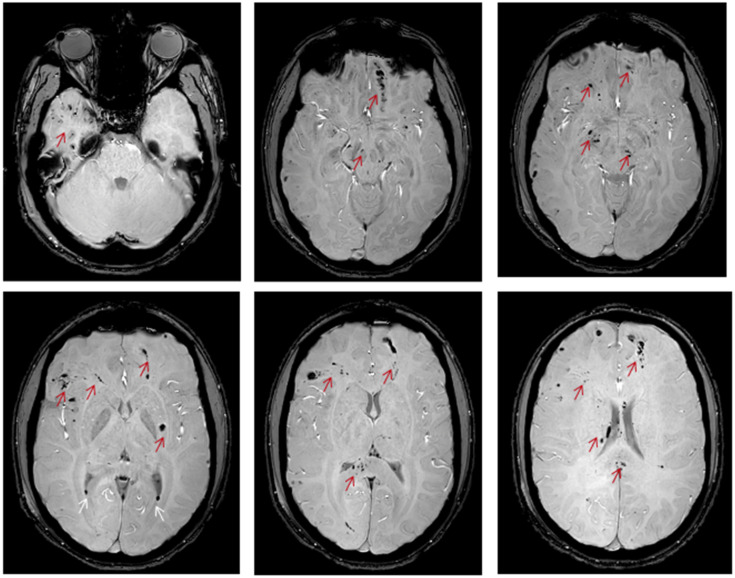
MRI of a 17-Year-old male with traumatic axonal injury, three days post traumatic brain injury (unpublished data), axial plane, SWI sequence shows multiple “dark spots’’ suggestive of haemorrhagic lesions mainly located in the gray–white matter junction of the right temporal, the fronto-occipital lobes and the right side of the splenium of corpus callosum, the midbrain and the left basal ganglia (red arrow). Key: MRI, magnetic resonance imaging; SWI, susceptibility weighted images.
